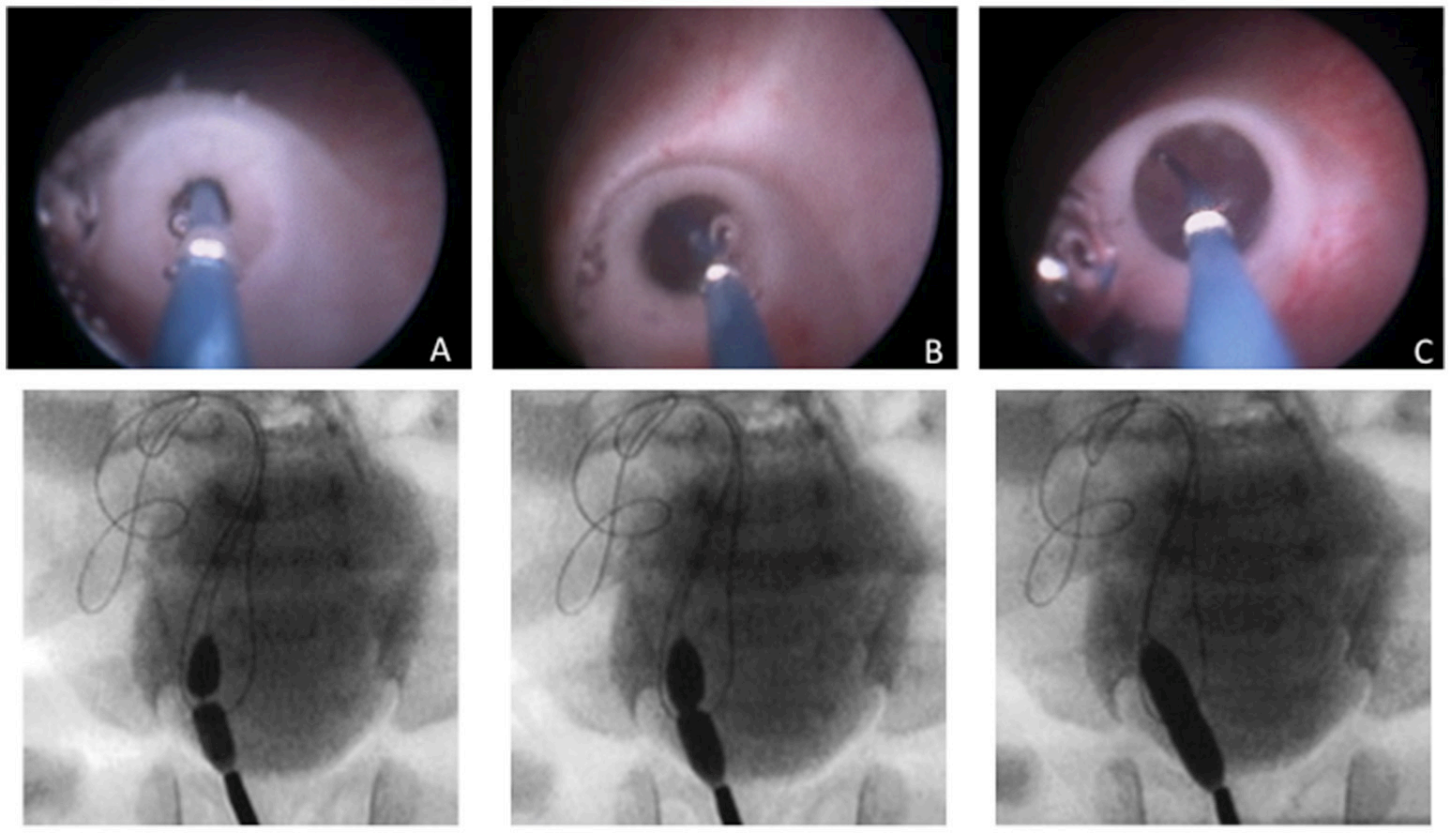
Balloon inserted through the right VUJ, endoscopic view and radiographic control during EBD procedure. (A) Initial balloon inflation with presence of stenotic ring; (B) Progressive dilation; (C) Complete expansion of the balloon and disappearance of the stenosis.
